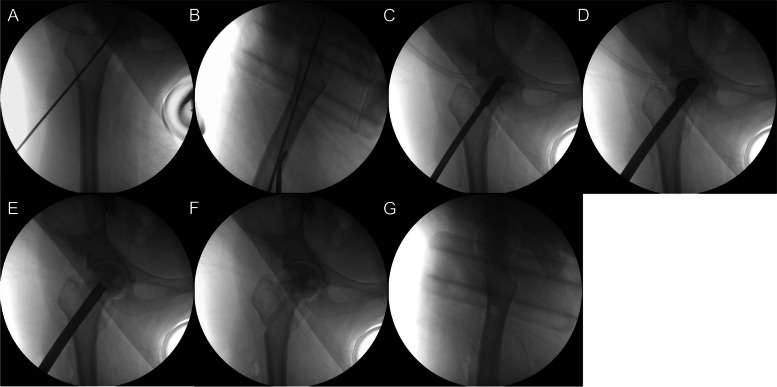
Intraoperative positioning by X-rays. The position of the guide wire was confirmed by anteroposterior (A) and lateral (B) X-rays. C A bone channel was reamed out over the K-wire to achieve core decompression. D Necrosis debridement was carried out with the reamer through progressive expansion of the blades. E The porous and dense granules previously mixed with bone sludge and pre-prepared PRP (Fig. 2) were grafted and impacted into the necrotic area. F and G The β-TCP rod was then inserted and implant positioning was confirmed by anteroposterior and lateral X-rays finally
